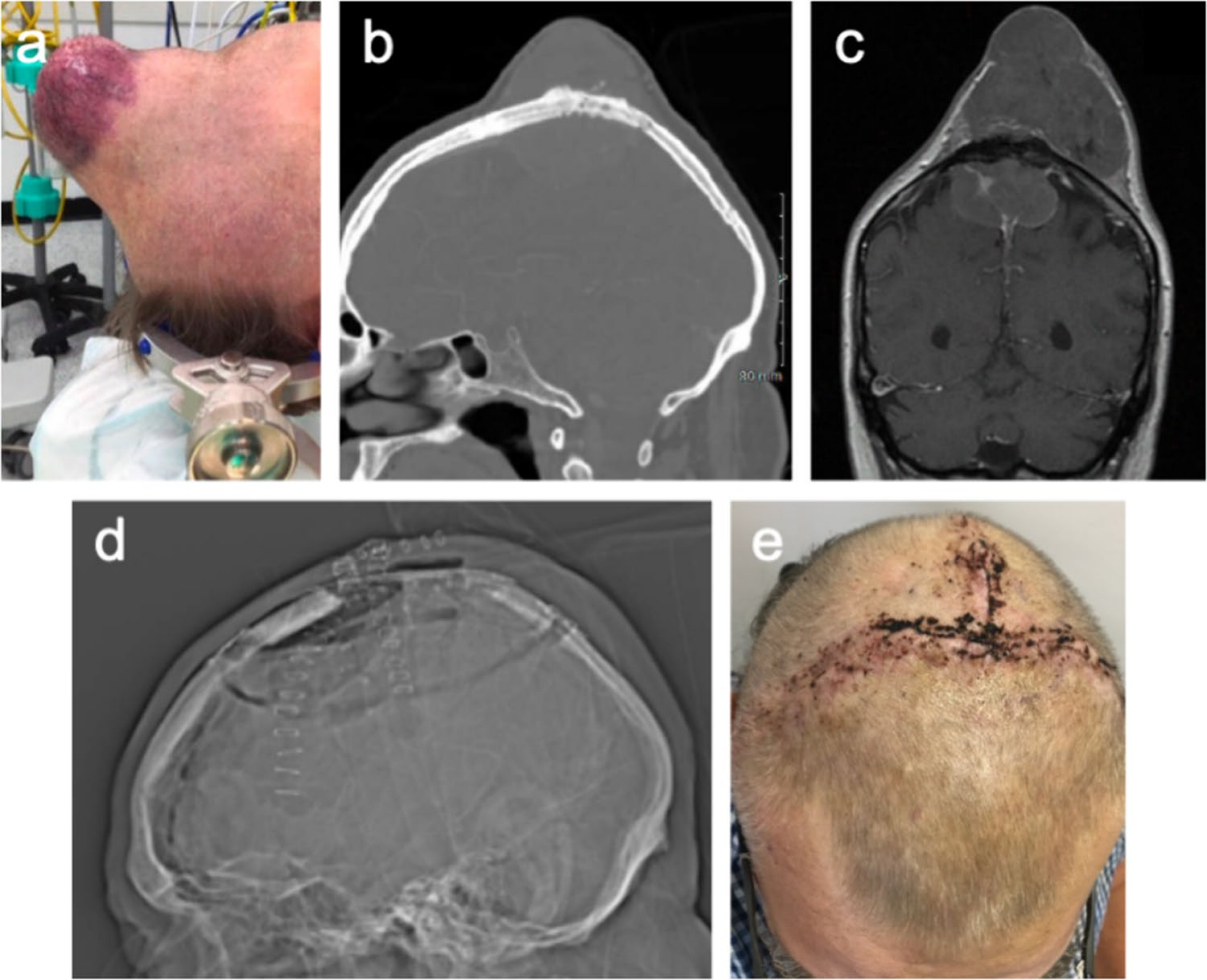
This 71-year-old man was reluctant to undergo treatment of his parasagittal meningioma, hiding under his hat a tumour that had grown through the bone and into the scalp. a, Preoperative photograph. b, Preoperative CT scan. c, Preoperative MRI scan. He underwent resection and reconstruction with EITC and primary skin closure in one sitting. The centre of the bone flap was replaced with tumour and was excised. This defect was covered with a titanium mesh. This was a CNS WHO grade 2 meningioma with Simpson grade 4 resection. The patient underwent external beam radiation therapy. Approximately 12 months after surgery the wound started to dehisce over the titanium mesh and the titanium was removed, which allowed the skin to heal. d, Post-operative CT scout image demonstrating bone and implant appearances. e, Post-operative skin appearances
